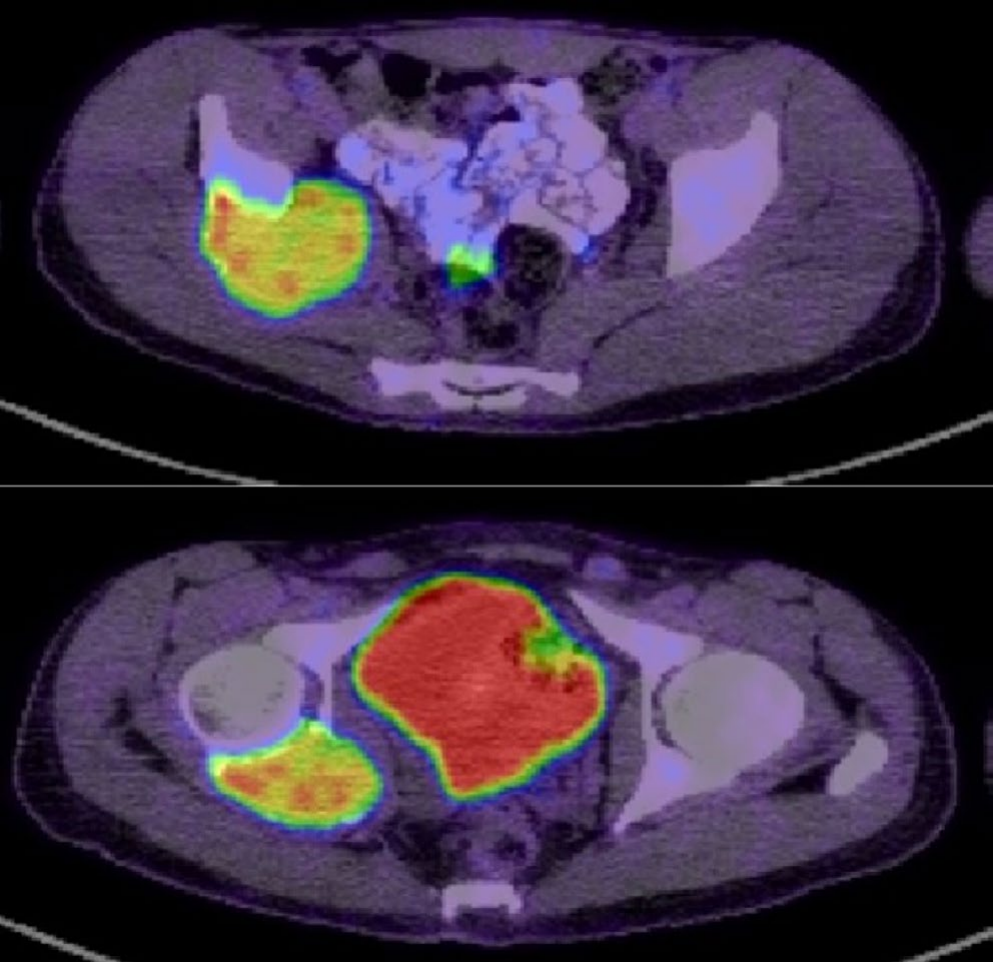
FDG PET/CT shows intensely increased tracer uptake in the expansile right acetabular/iliac osseous lesion.
